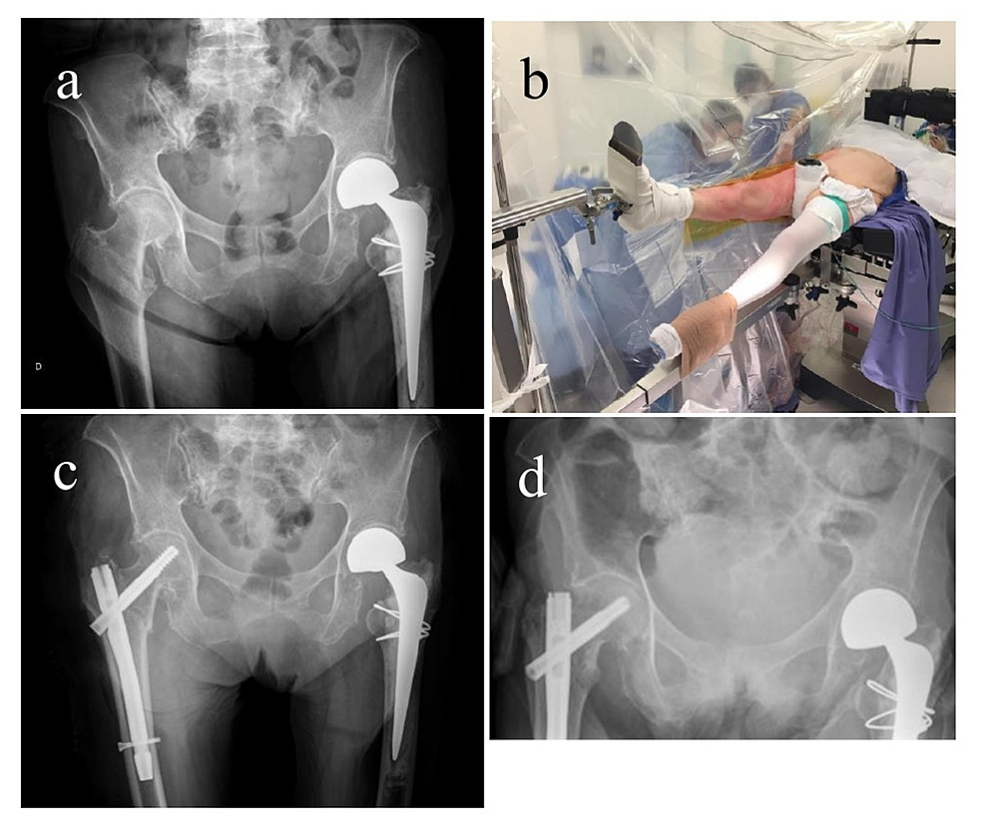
Left hip hemiarthroplasty and right intertrochanteric fracture (a) An 81-year-old female patient; preoperative anteroposterior pelvic radiographs demonstrating a history of left hip hemiarthroplasty and a right intertrochanteric fracture. (b) Example of the scissor position on a fracture table using a small pad and a bandage to protect and secure the foot of the well leg. (c) Immediate postoperative pelvis X-ray and (d) 10-month follow-up X-ray.
